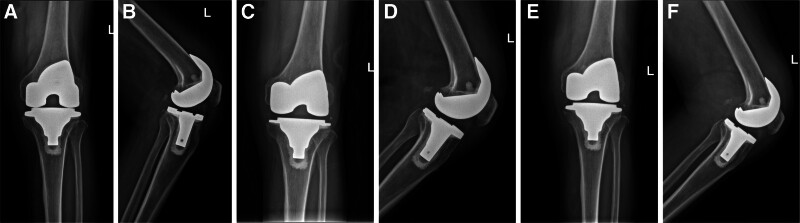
(A–F) radiographic progression of the left knee: (A) postoperative 37 d anteroposterior projection radiograph; (B) Postoperative 37 d lateral projection radiograph; (C) Postoperative 9 mo anteroposterior projection radiograph; (D) postoperative 9 mo lateral projection radiograph; (E) postoperative 16 mo anteroposterior projection radiograph; (F) postoperative 16 mo lateral projection radiograph.
